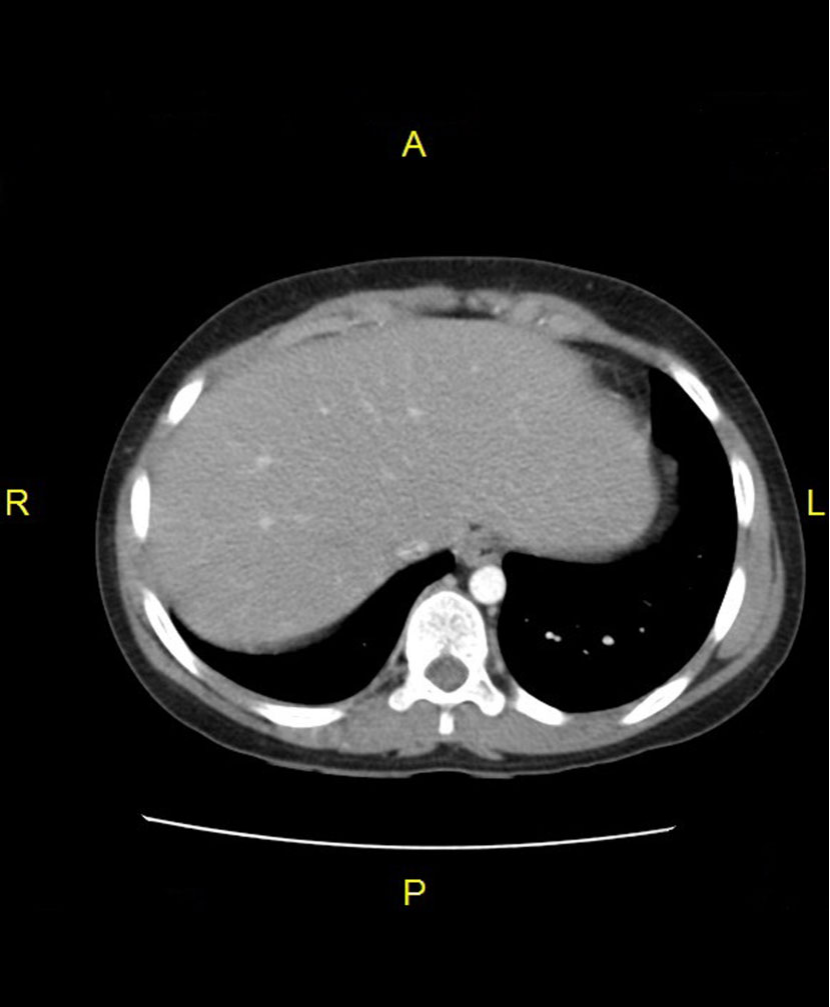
Liver CT after treatment.
